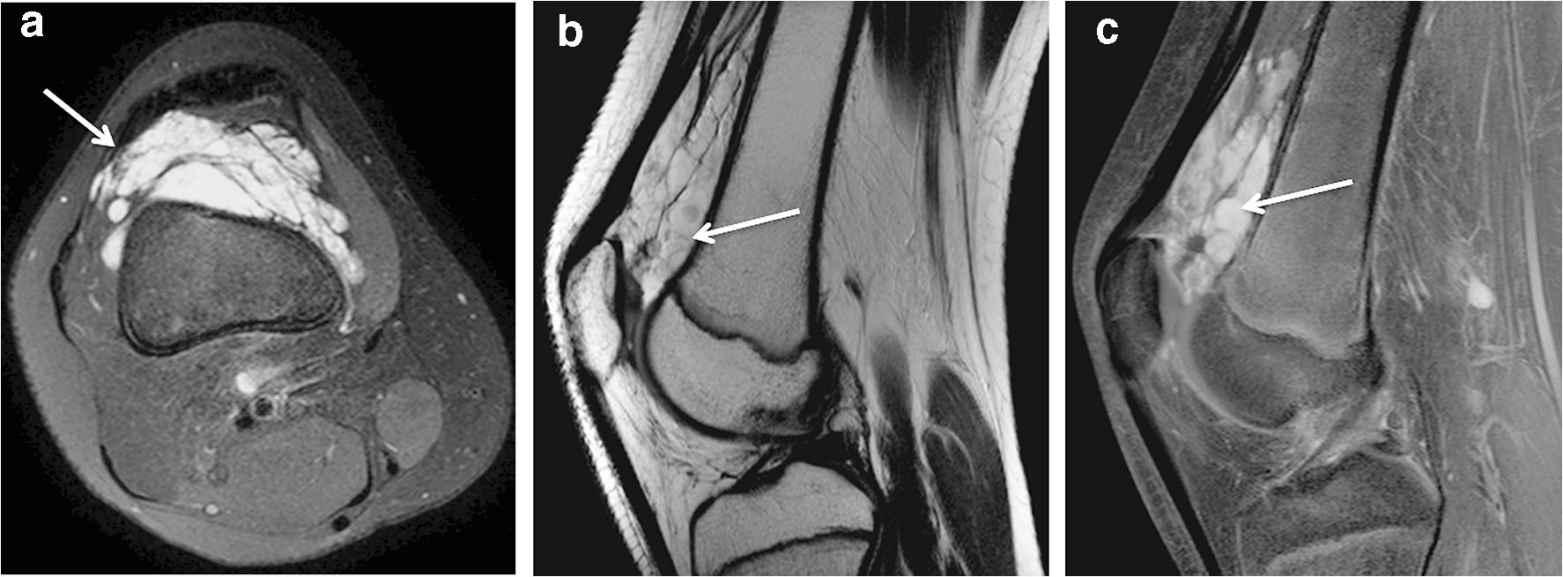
A 12-year-old girl with a venous malformation of the suprapatellar fat pad. a A T2-weighted fat-saturated axial MRI shows a typical cluster-like lesion consisting of tubular structures (*arrow*). b A T2-weighted sagittal MRI demonstrates the close proximity of the suprapatellar synovial recess and the venous malformation. The outline of the synovium is not identifiable between the venous malformation and femoral cortex (*arrow*) and possible synovial involvement cannot be ruled out. c A T1-weighted fat-saturated MRI shows partial enhancement of the lesion after gadolinium injection (*arrow*)
